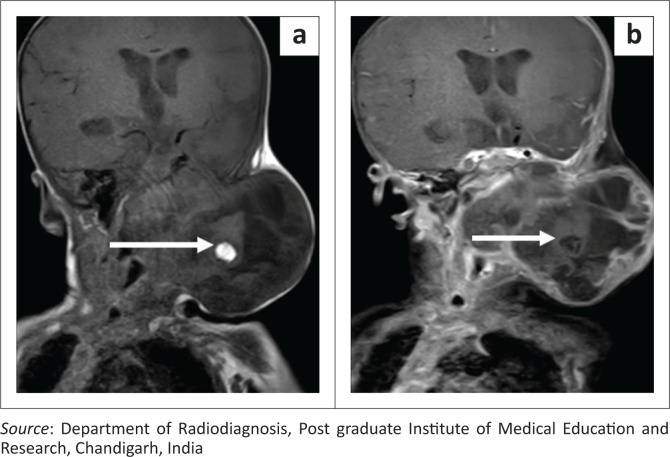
Coronal T1-weighted pre-contrast image (a) demonstrates a predominantly T1-hypointense cystic component, with the solid component iso to hypointense in signal to brain grey matter. A small central focus of intrinsic T1 hyperintensity (arrow) is noted within the lesion. On the coronal post-contrast fat-suppressed T1-weighted image (b), the solid component and septa reveal enhancement, while the central T1-hyperintense focus (arrow) demonstrates signal suppression, likely representing fat.
